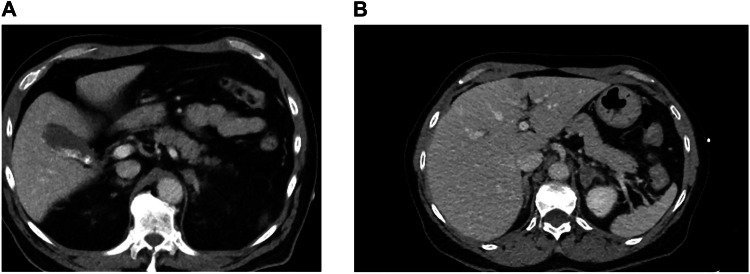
A CT imaging of an adrenal lesion in a patient at the initial trauma CT. A left adrenal lesion of 17 mm with an attenuation of up to 52 HU in a middle-aged woman. The left adrenal was mentioned as prominent in the initial report. No follow-up CT was performed. B CT performed 2 years after the trauma CT in the same patient. However, a contrast enhanced CT under another question two years later showed the lesion with similar size and a HU of 52
